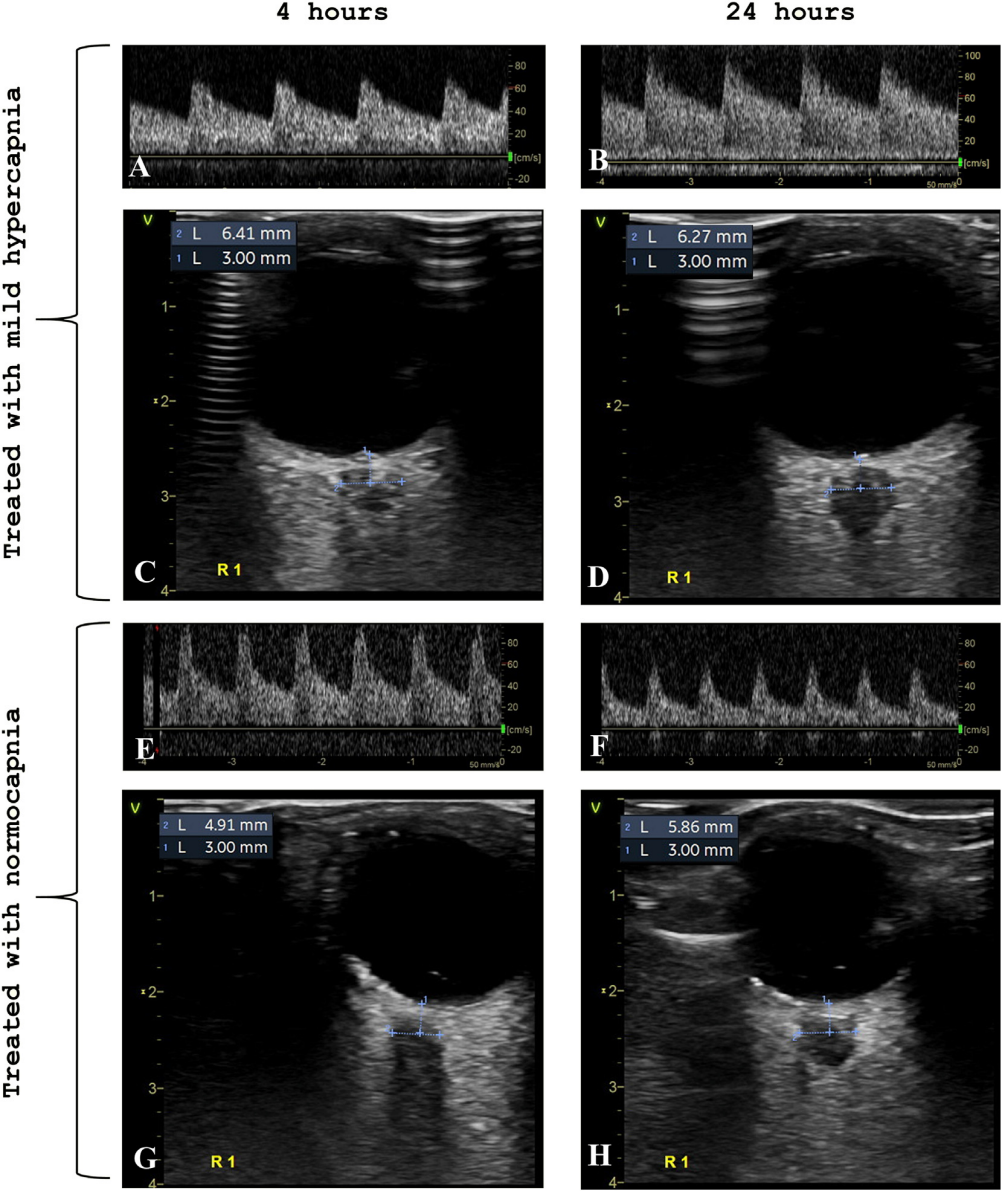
Title: Representative images of transcranial Doppler and transorbital ultrasound at 4 and 24 h for at surviving and a non-surviving patient. Legend: Representative ultrasound images for a survivor (A–D) and a non-survivor (E–H). For the survivor, MCA PI (A and B) and ONSD (C and D) decreased from 4 to 24 h. For the non-survivor, MCA PI (E and F) and ONSD (G and H) increase from 4 to 24 h. MCA PI is defined as the difference between peak systolic velocity and end diastolic velocity divided by mean velocity in a cardiac cycle. The indexing makes the parameter independent of the angle of insonation. MCA PI: middle cerebral artery pulsatility index. ONSD: optic nerve sheath diameter.
